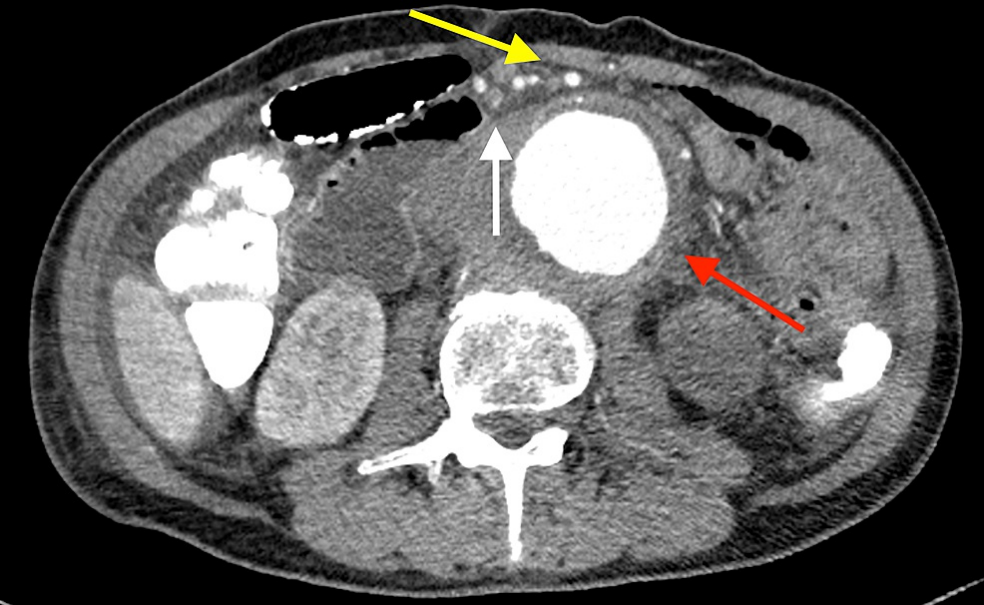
Abdominal CT. The duodenum (white arrow) is compressed between the superior mesenteric artery (yellow arrow) and abdominal aortic aneurysm (red arrow). CT: computed tomography
